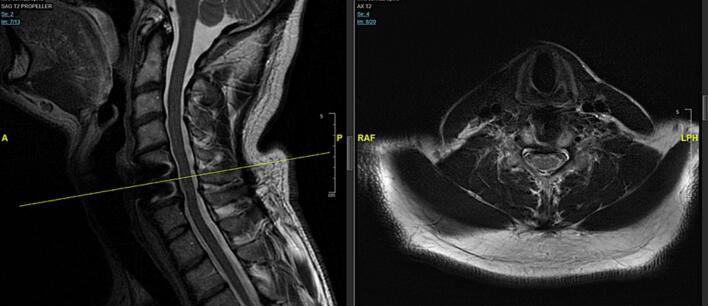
Follow-up sagittal T2-weighted MRI of the cervical spine obtained 3 years and 6 months postoperatively, demonstrating solid fusion at the C5–C6 level with no recurrent disc herniation or spinal cord compression.
